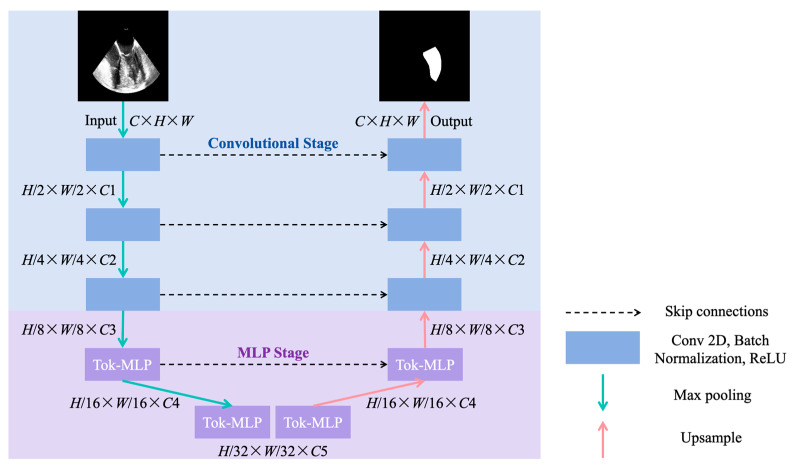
Structure of UNeXt [26]. (*C*, *H*, *W*) represent the channels, height, and width of the TEE image, respectively. Conv: convolutional; ReLU: rectified linear unit; MLP: multilayer perceptron; Tok-MLP: tokenized multilayer perceptron; TEE: transesophageal echocardiography.
